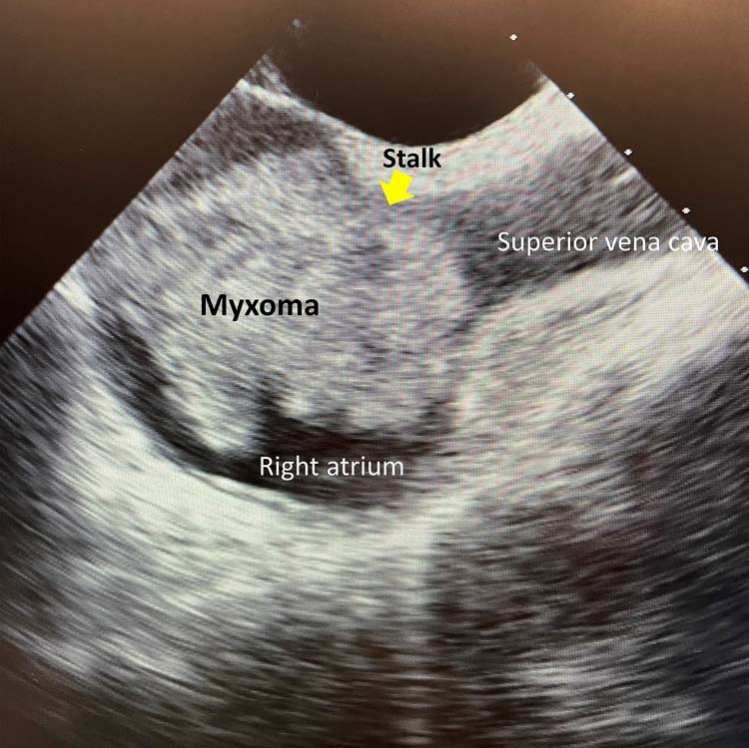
Preoperative transesophageal cardiac echocardiography.
